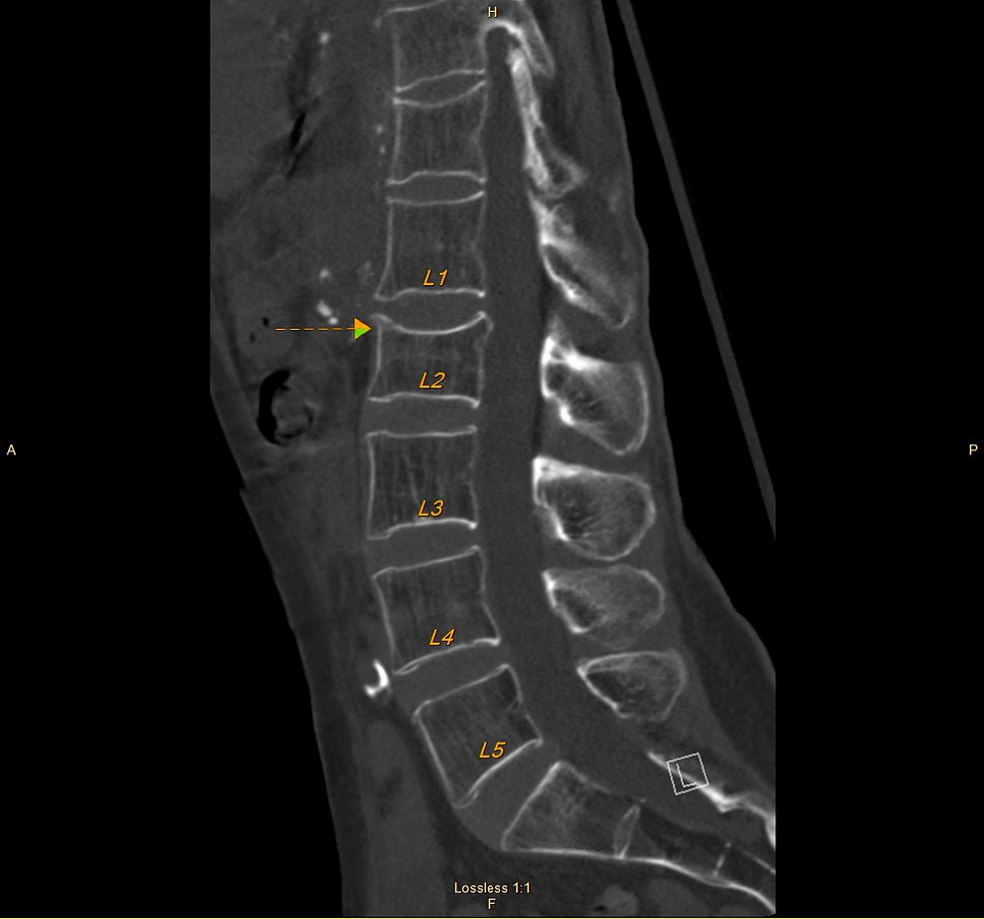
NCCT image of the lumbar spine: sagittal view The image shows an incidental finding of anterior step defect with a vague line of condensation just inferior to this consistent with a subacute L2 compression fracture NCCT: non-contrast computed tomography; L2: second lumbar vertebra
